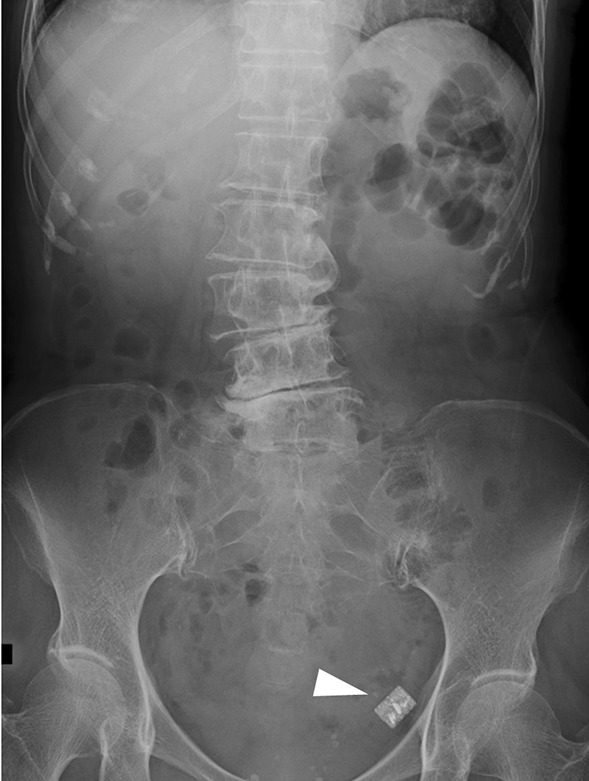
Abdominal X-ray showed the patency capsule (arrow head) remains in the small intestine
